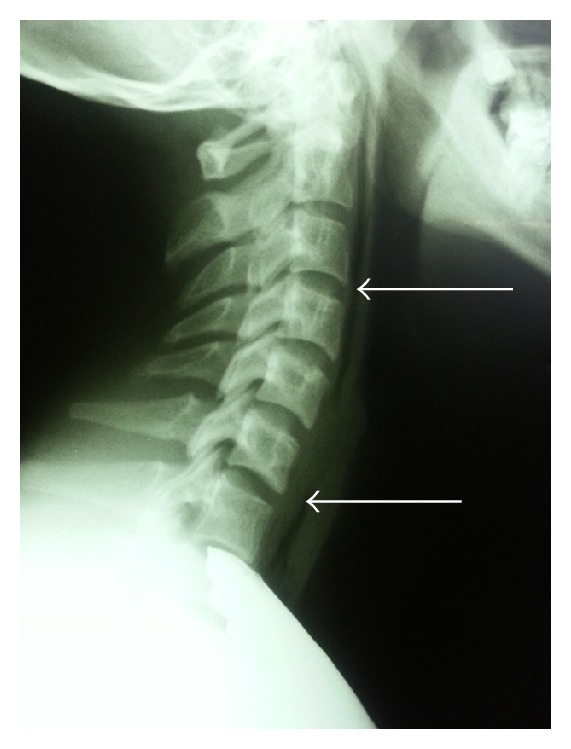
Neck X-ray, lateral view; free air is indicated by arrows.
